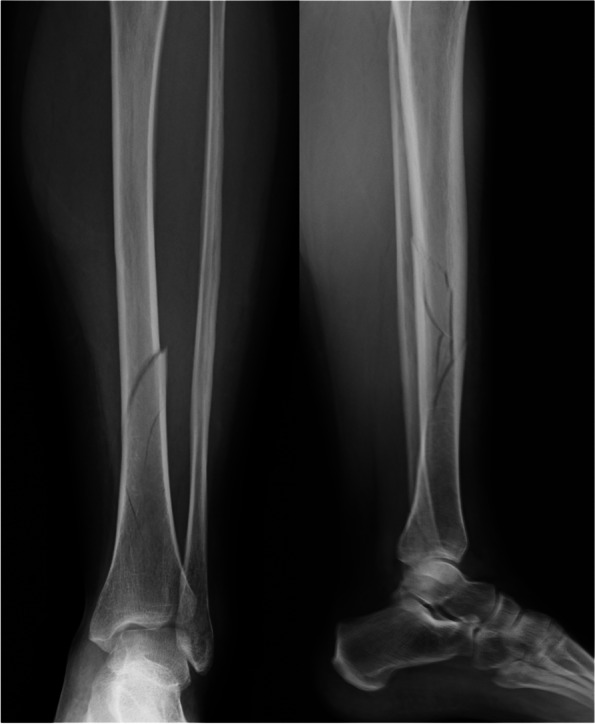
Preoperatory X-ray of the left leg
